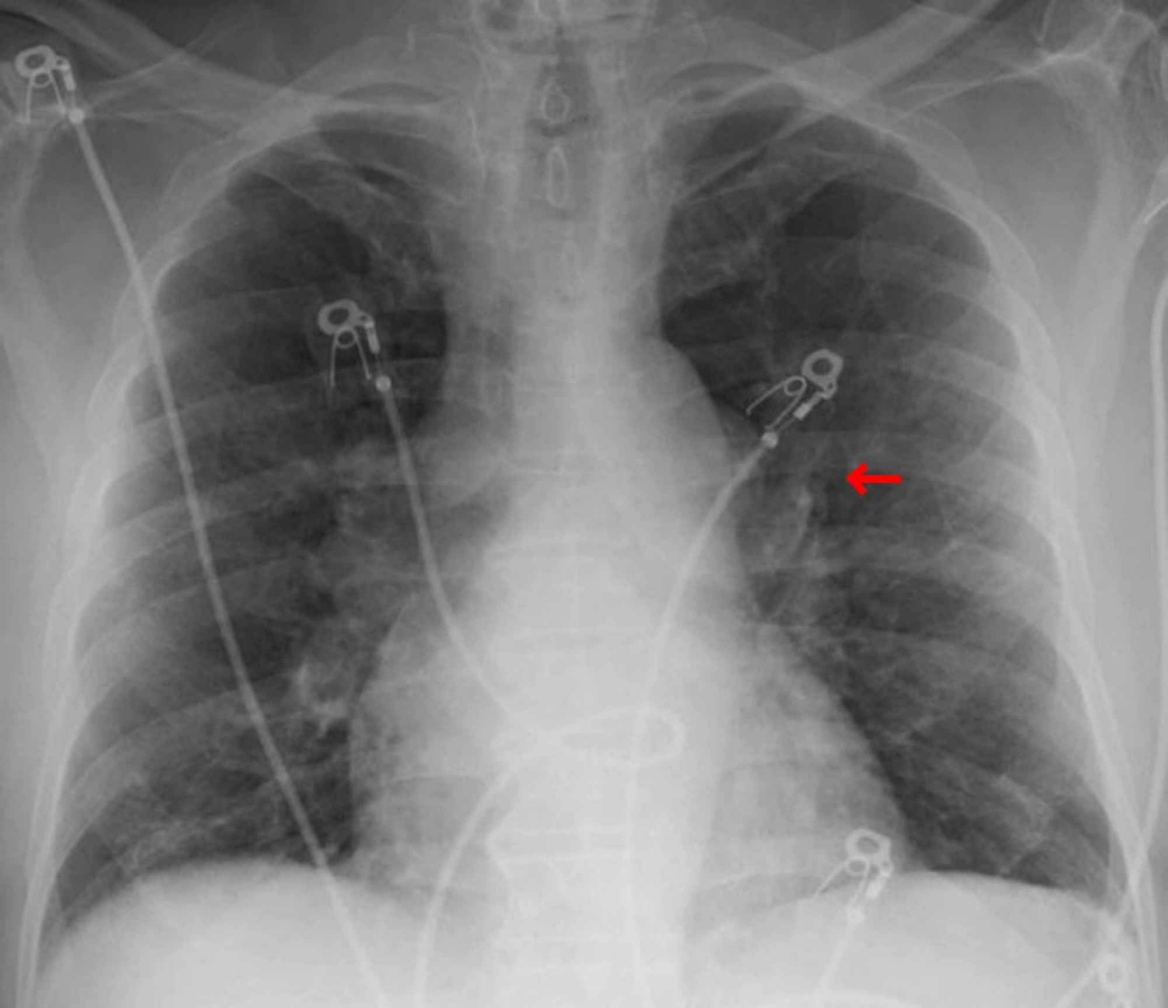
Patient's chest X-ray which appears generally clear with faint opacity in the left perihilar region (red arrow).
